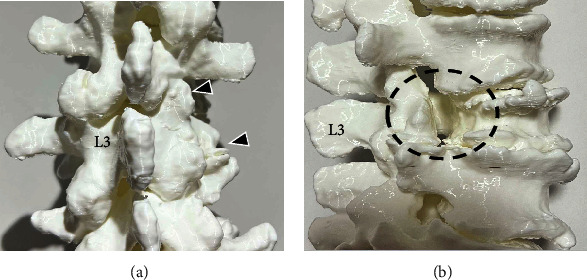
Preoperative computed tomography–based 3-dimensional bone model. (a) Dorsal and (b) right lateral views. Arrowheads show hypoplasia of the right L2 lower articular process (lower) and the right L3 transverse process. Black dotted line shows absence of the right L3 pedicle.
